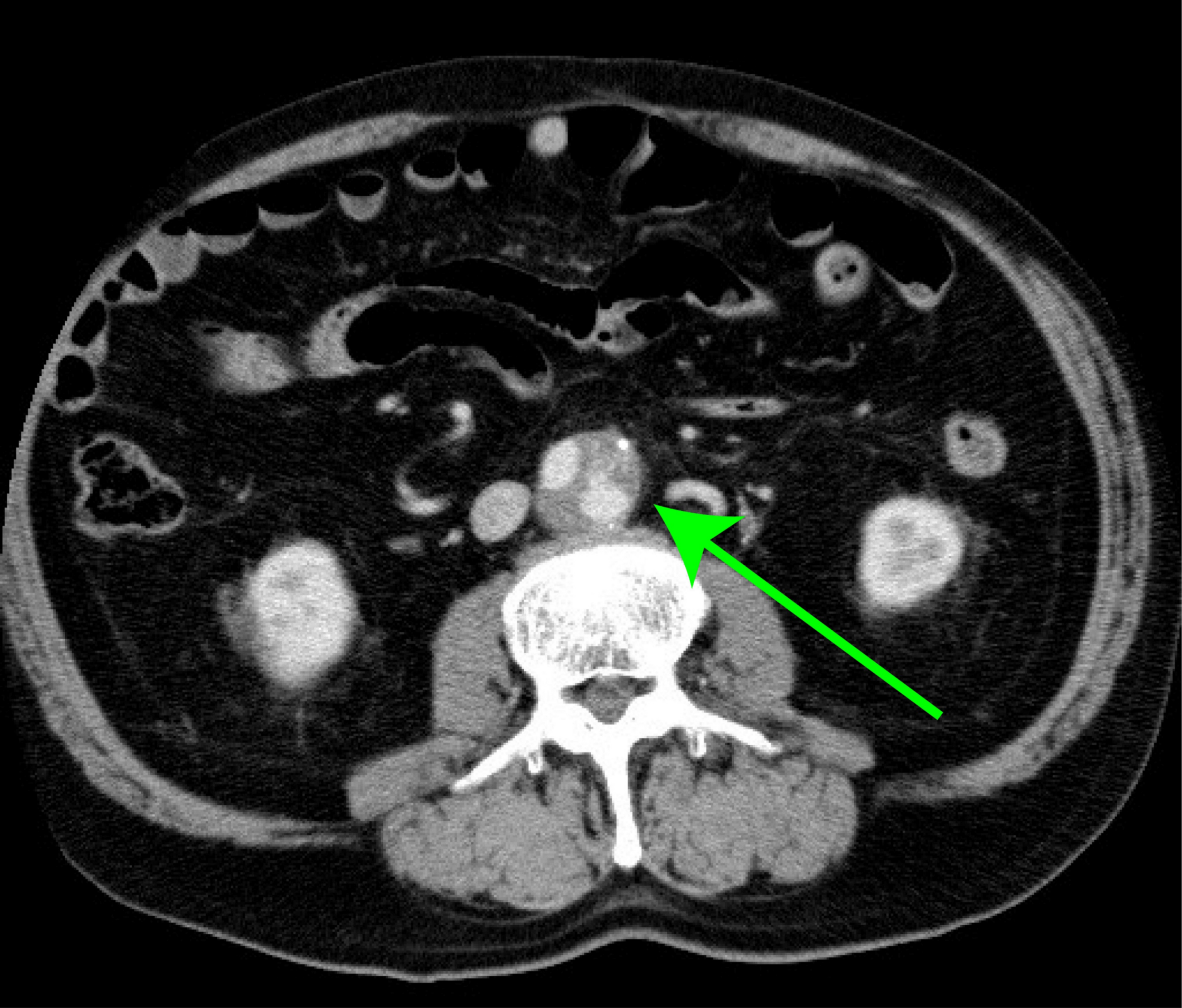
Preoperative computed tomography image. Contrast-enhanced computed tomography image taken at 25 years post-implantation revealing the synthetic graft within the aortic sac containing contrast leakage (green arrow).
